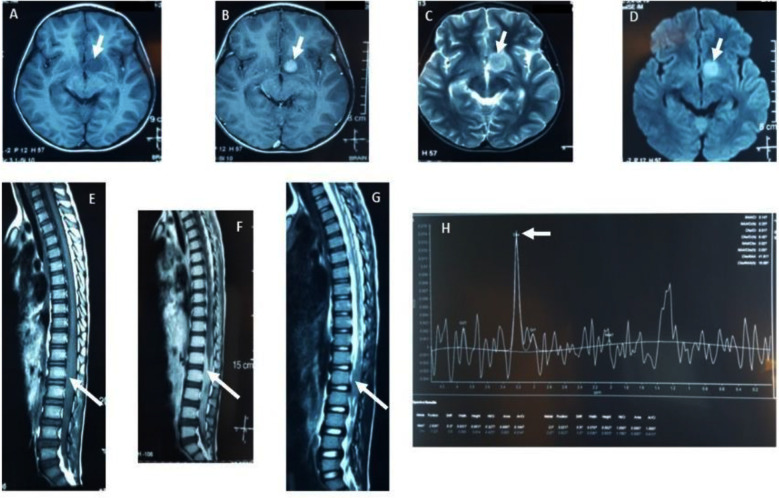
Neuroimaging of one of the patients with a primary left deep frontal lobe tumor and spinal metastasis (white arrows point out the lesions in the brain and the spinal cord). A: T1W Axial image, Brain: Hypo-intense lesion in left deep frontal lobe; B: T1W post-contrast axial image, Brain: Contrast-enhanced lesion in left deep frontal lobe; C: T2W Axial image, Brain; D: FLAIR Axial image, Brain; E: T1W sagittal image, whole spine: Diffuse soft tissue thickening around the terminal spinal cord in the spinal canal; F: T1W post contrast sagittal image, whole spine: Contrast enhancement in the terminal spinal cord; G: T2W image, sagittal image, whole spine: Hypo-intense lesion in the terminal spinal cord and H. MRS: Raised choline peak.
